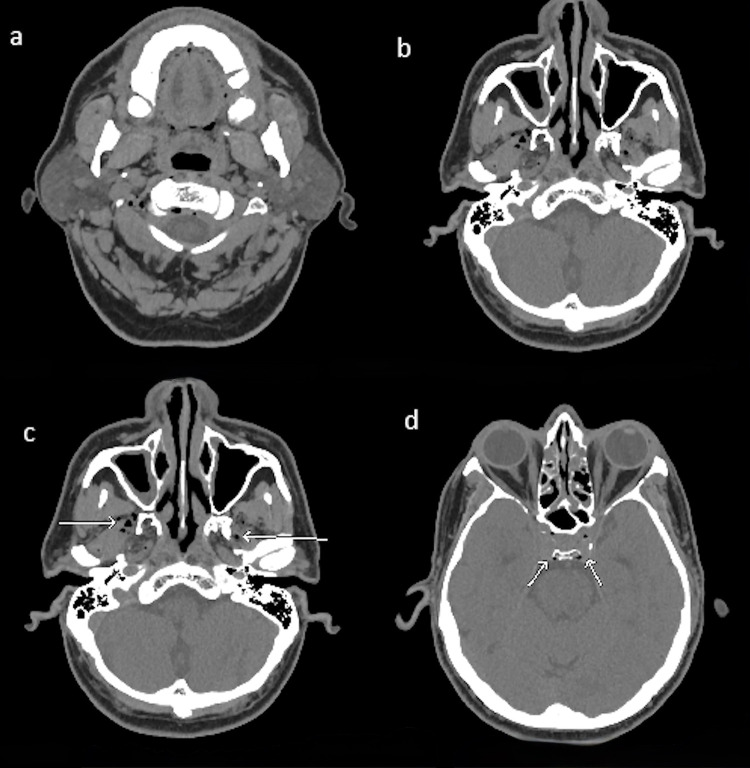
Initial non-contrasted computed tomography (CT) of the head and upper neck demonstrating venous air. (a) Axial section through the upper neck revealing small air foci in within the retro-dental soft tissues posterior to odontoid process, and the right paravertebral region venous plexus. (b and c) Axial images at the skull base showing air specks within the bilateral pterygoid venous plexuses (white arrow). (d) Axial bone window at the level of cavernous sinuses showing small air foci within both cavernous sinuses (white arrow).
